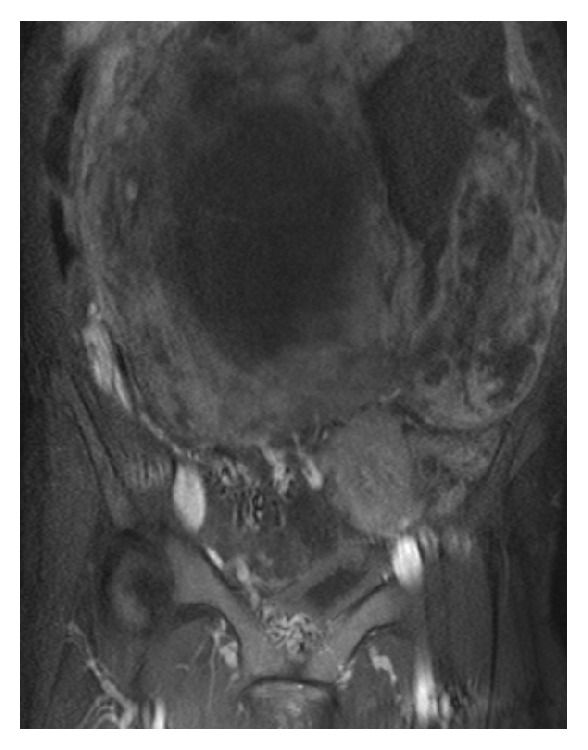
MRI image of a Gadolinium enhanced T1-weighted coronal section. The center of the abdominal tumor was not enhanced, implicating a suspicion of necrosis in the center.
